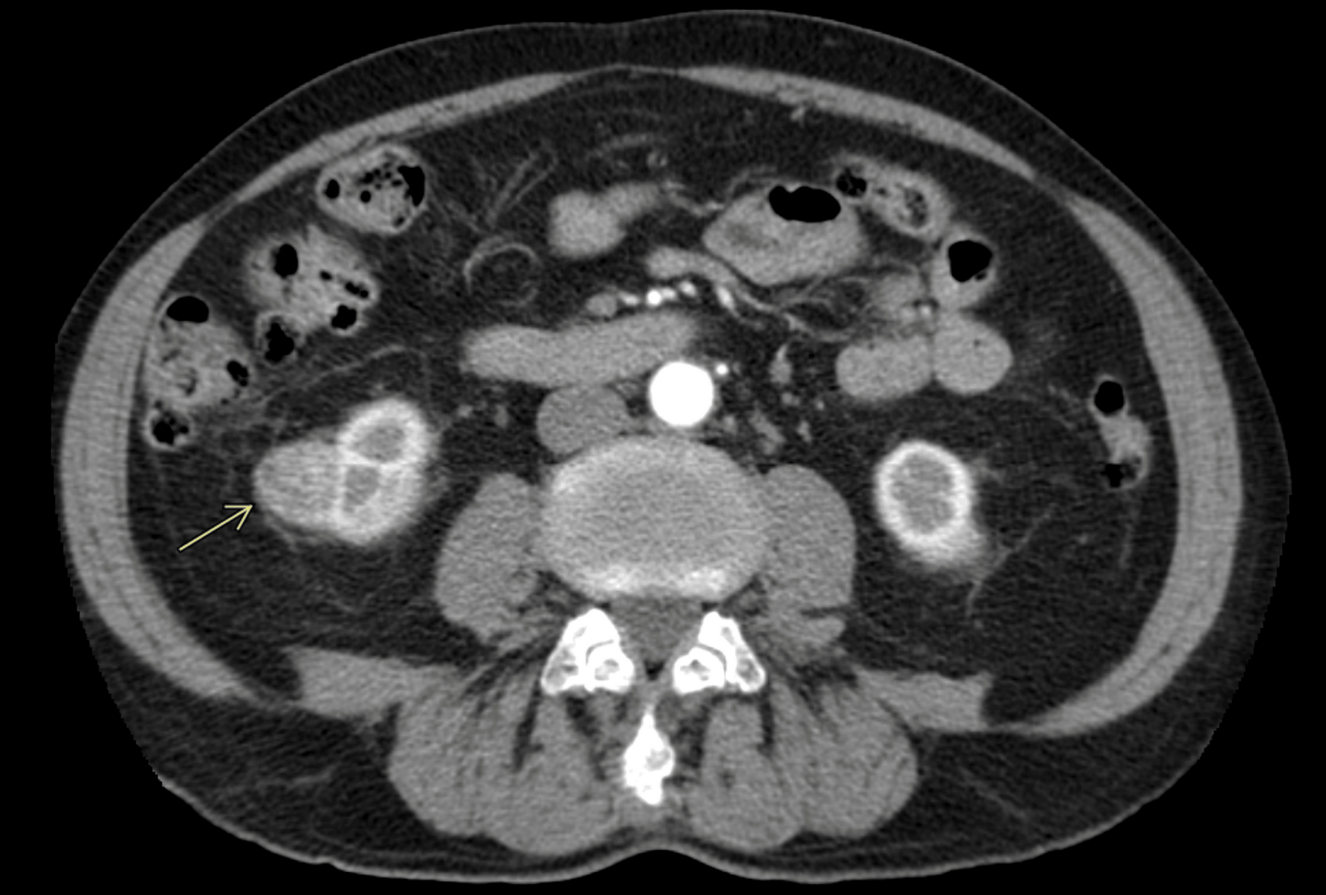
Axial abdomen and pelvis CT scan with IV contrast/abdomen window showing a 2.0 x 2.3 cm exophytic right lower pole heterogeneously enhancing lesion consistent with an oncocytoma The arrow points to the kidney lesion
